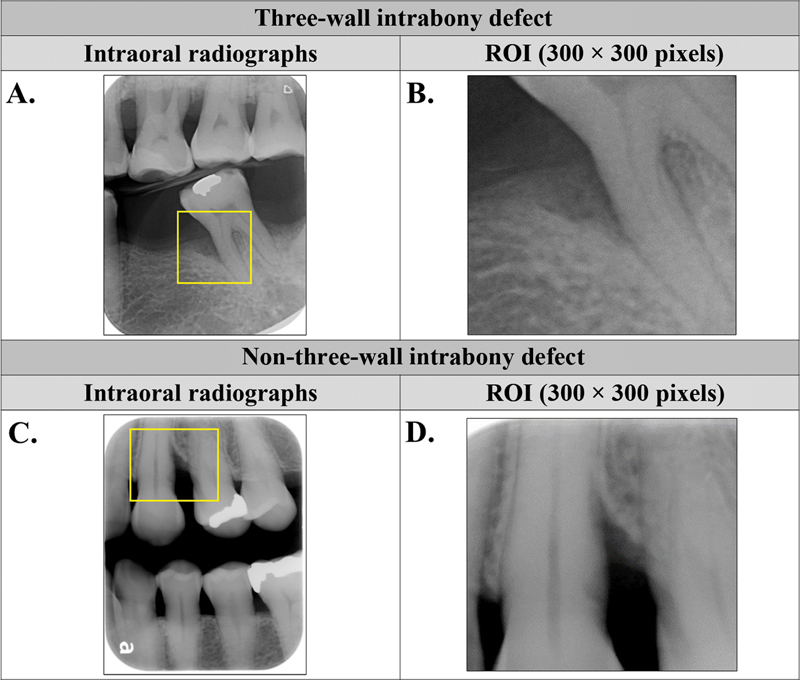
Images demonstrated representative vertical bitewing radiographs, which categorized into three-wall (2A, B) and non-three-wall bony defects (2C, D). Original images (2A, C) were cropped into the ROI (yellow rectangular box) at 300 × 300 pixels (2B, D). ROI, region of interest.
